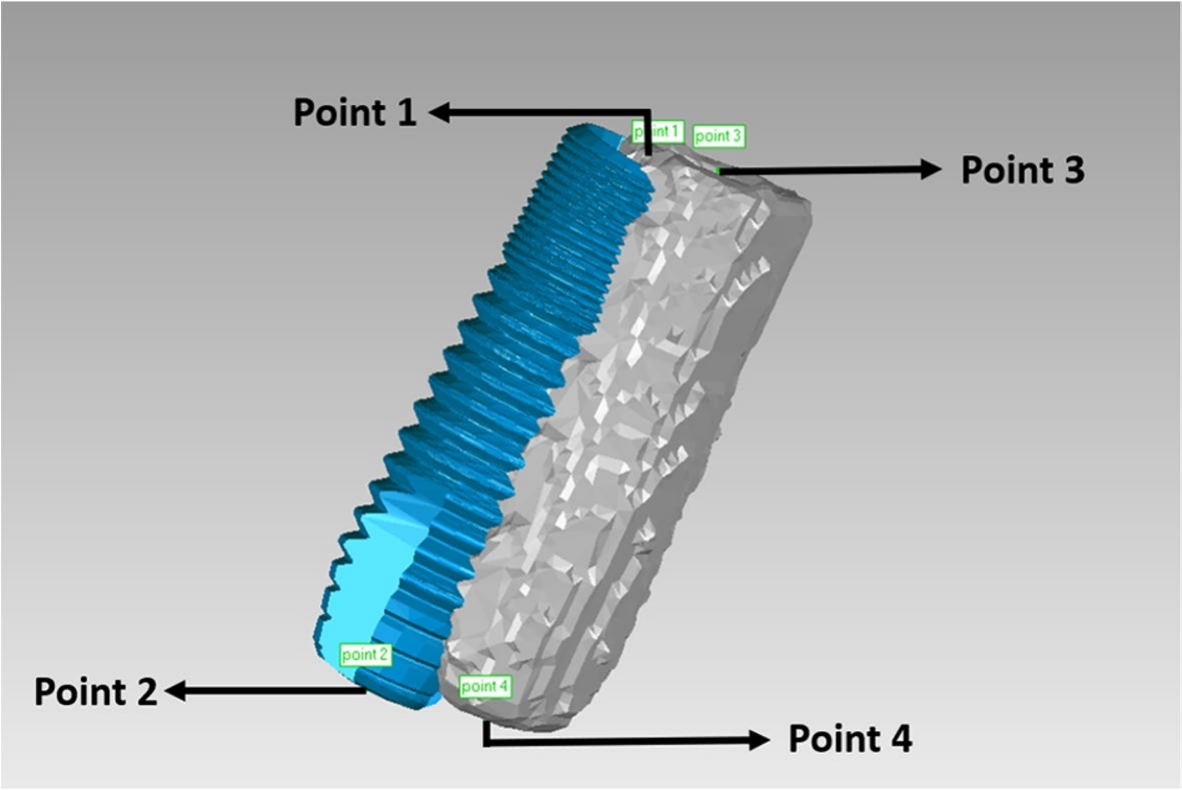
The positional relationship between the planned (blue) and actual (grey) implant sites was determined by the conventional radiographic method. (Point 1: the entry point of the planned implant; Point 2: the apex point of the planned implant; Point 3: the entry point of the actual implant determined by the conventional radiographic method; Point 4: the apex point of the actual implant determined by the conventional radiographic method)
